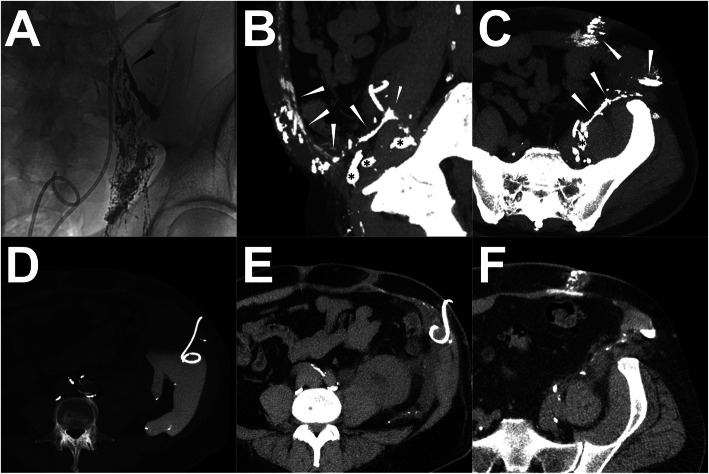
A patient underwent a DLS procedure after post-LAG CT. Note: Conventional iodized oil-based LAG showed the extravasation (black arrowhead) from the left iliac LVs (a). From the sagittal (b) and axial MIP (c) images of post-LAG CT is demonstrated an obvious LF (white arrowhead) from the iliac LVs into the peripheral lymphocele around the transplanted kidney. The afferent LNs could be identified (*). A pigtail drainage catheter was inserted into the lymphocele before the local sclerotherapy (d). After running out of all the lymphatic fluid, 15 ml of 95% Ethanol as the sclerosant was injected to flush the lymphocele and then this was drawn out twice. Eight (8) days after DLS, the CT scan revealed very limited residual lymphatic fluid (e, f). Abbreviations: DLS – direct leakage sclerotherapy; post-LAG CT – post-lymphangiographic computed tomography; LAG – lymphangiography; LVs – lymphatic vessels; MIP – maximum intensity projection; LF – lymphatic fistula; LNs – lymph nodes
